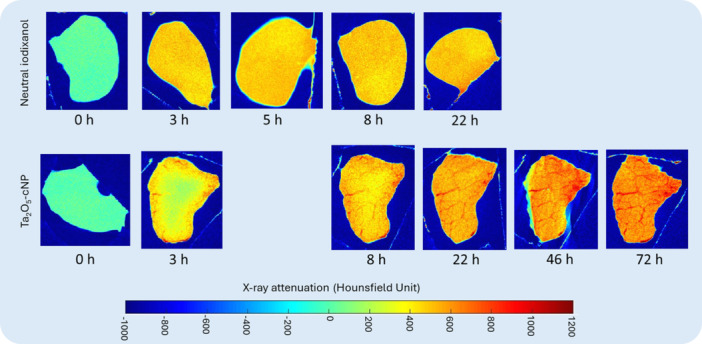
Two‐dimensional CT images (by averaging 10 consecutive slices = ~170 µm) of ligament samples immersed in neutral iodixanol (top row) and Ta_2_O_5_‐cNP (bottom row) acquired at each timepoint. Initial bath attenuations are shown on the right. The slices were selected from the central region of the sample. As the samples were not co‐registered between timepoints, the representative slice might not be from the exact same location at each timepoint.
